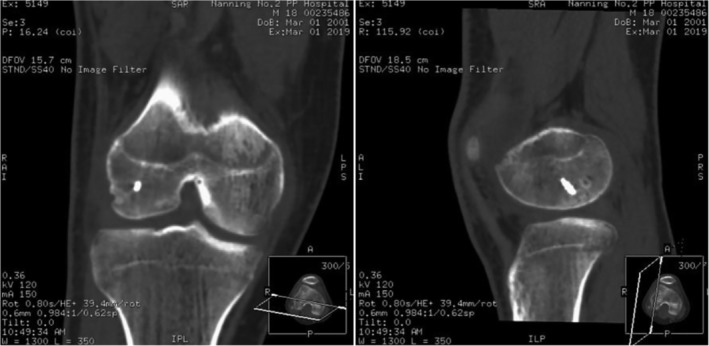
CT examination 6 months after operation: one screw internal fixation, regular external condyle cortex, good alignment at the end of fracture, callus growth and unclear fracture line could be seen in the right lateral femoral condyle.
